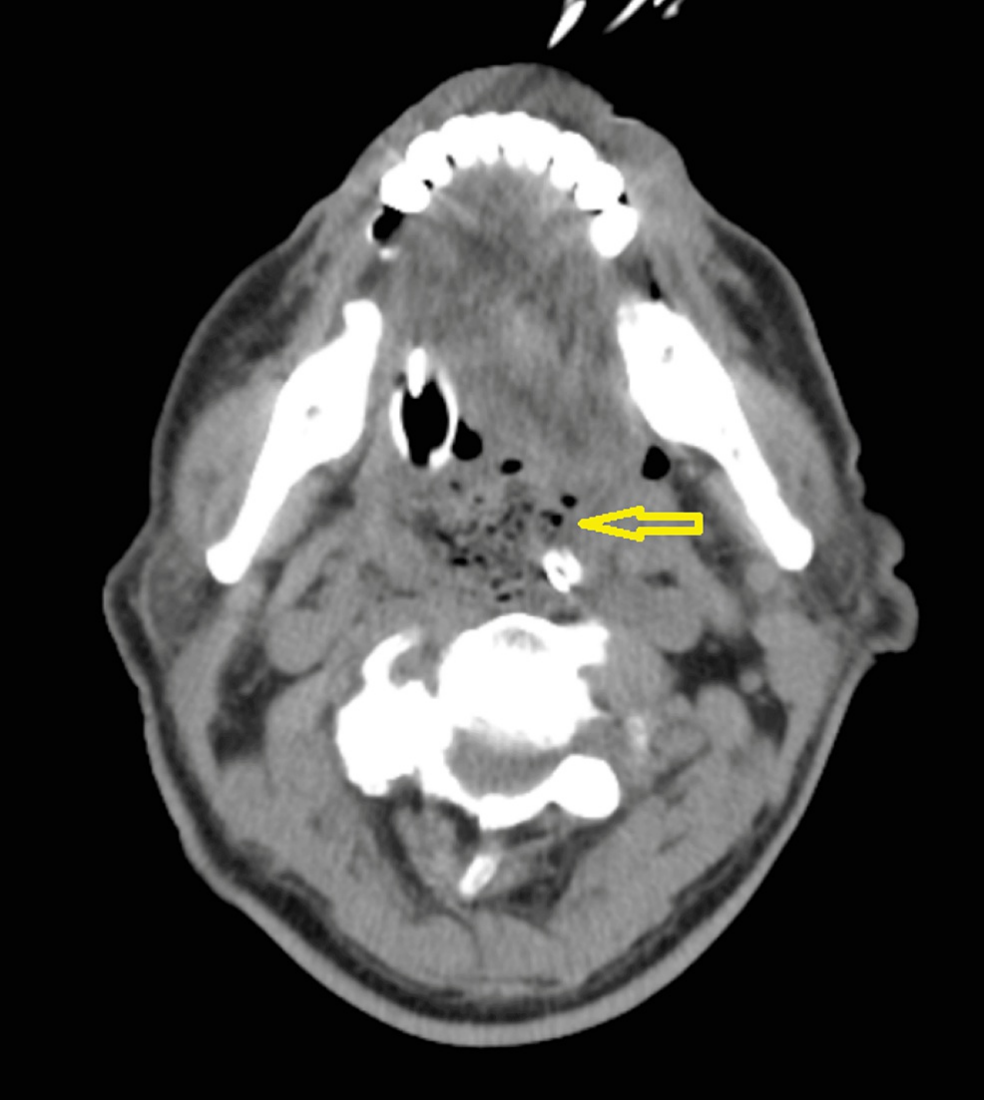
Computed tomography scan of the neck. Transverse section showing 3.1 × 3.3 cm abnormal soft tissue density and air density opacifying the oral pharyngeal and hypopharyngeal airway.
